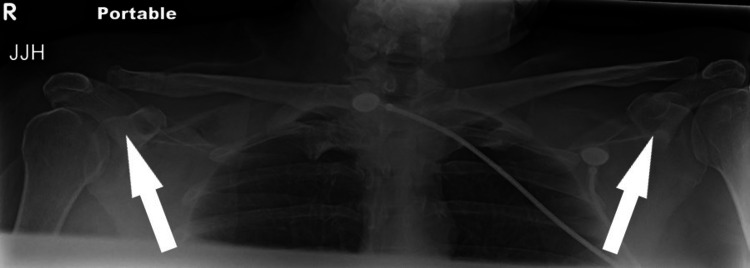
Bilateral anterior-posterior (AP) X-rays after removal of hardware at four months postoperatively
